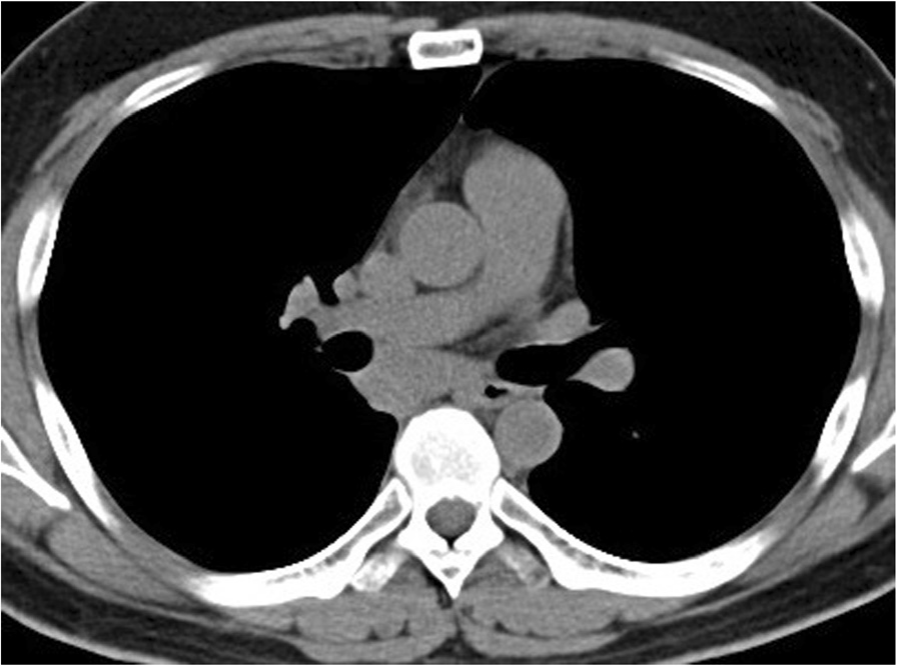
Computed tomography scan after 14 months postoperatively showing no recurrence of MBC
